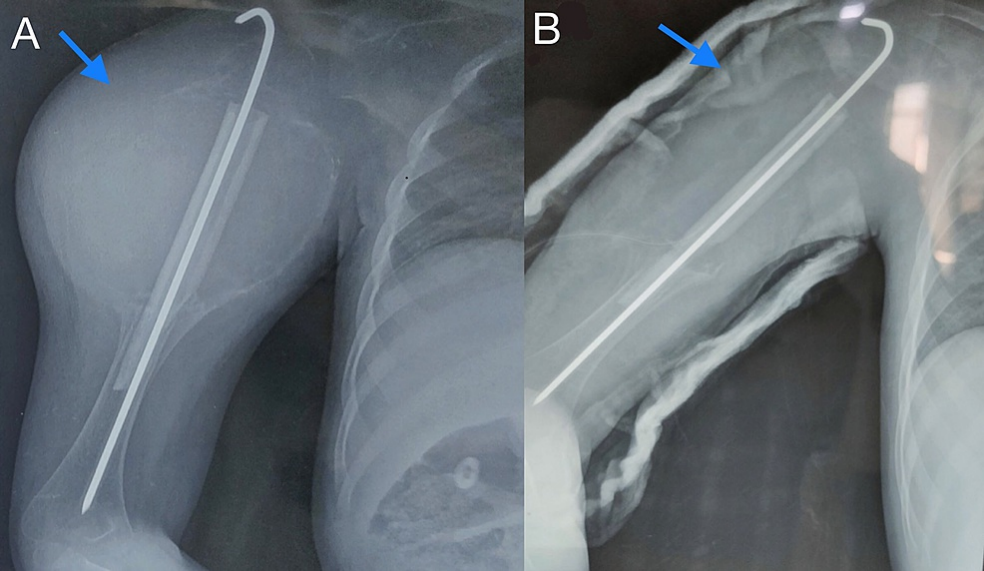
Preoperative (A) and postoperative (B) plain radiograph at 10-month follow-up showing excision and curettage of the tumor followed by chemical cauterization.
